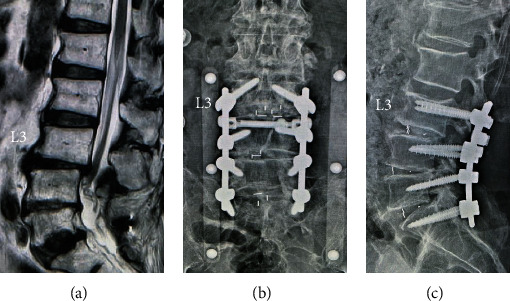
(a) Sagittal T2-weighed magnetic resonance imaging 2 years after primary surgery showing posterior spondylolisthesis, bulging disc, and canal stenosis at L3-4. (b, c) Postoperative radiographs after additional posterior fusion surgery at L3-4.
